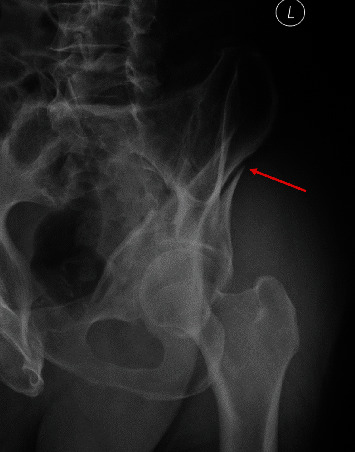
Radiographic characteristics of both-column fractures with PW involvement. The “antispur” sign is marked with a red arrow in the obturator-oblique view.
